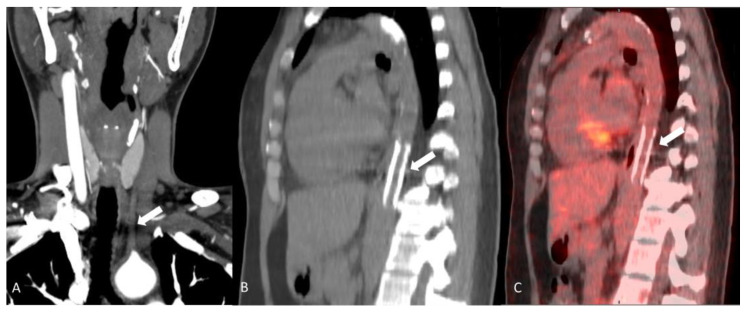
A 32-year-old woman with history of Takayasu’s disease. Coronal maximum intensity projection image of neck CTA (A) showing complete occlusion to the left common carotid artery (arrow). Sagittal images of CT without contrast (B) and F-18 FDG PET/CT (C) demonstrating distal descending aorta stent (arrows) with no pathological abdominal wall radiotracer accumulation consistent with sequela of chronic arteritis with no evidence of acute inflammation.
